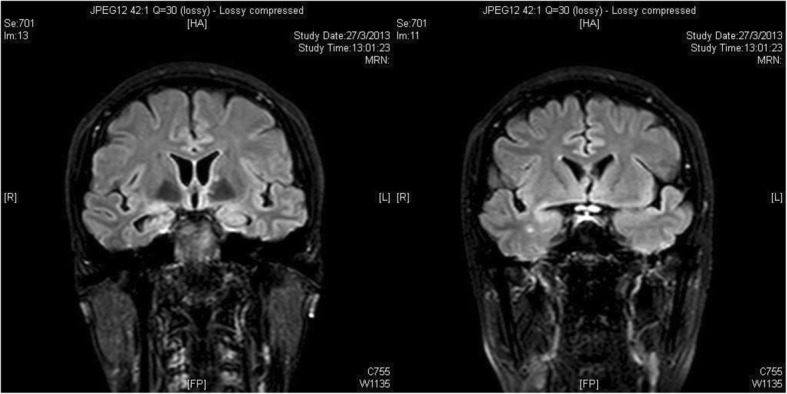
Magnetic resonance imaging of the brain with contrast performed on 27 March 2013. Abnormal T2-weighted (T2W) hyperintense signal is seen along the bilateral amygdala and hippocampi, resembling limbic encephalitis. Bilateral optic nerves are enlarged, exhibiting T2W hyperintensity with contrast enhancement compatible with optic neuritis
